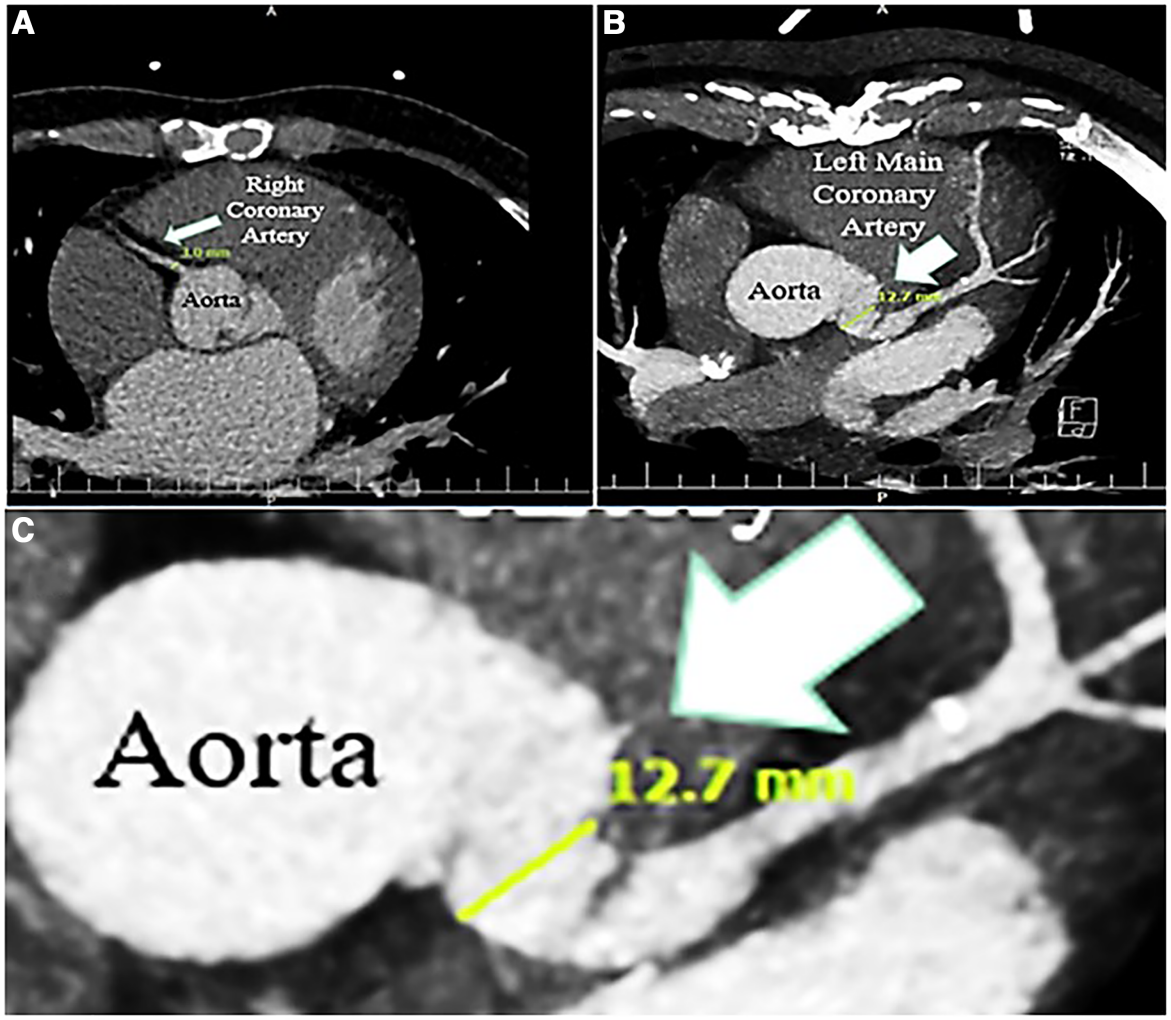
Contrast-enhanced chest computed tomography scan, showing normal caliber right coronary artery (3 mm) (A), and dilated left main coronary artery (12.7 mm); zoom out (B), and zoom in (C).
